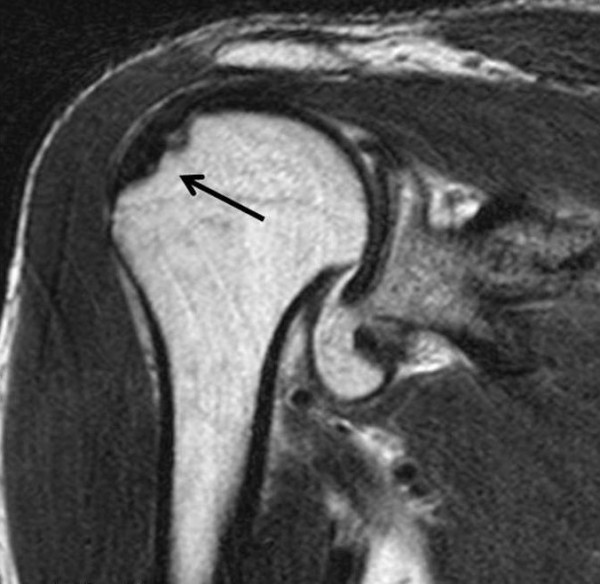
MRI showing Hill-Sachs variant on superolateral aspect of humeral head(arrow).
